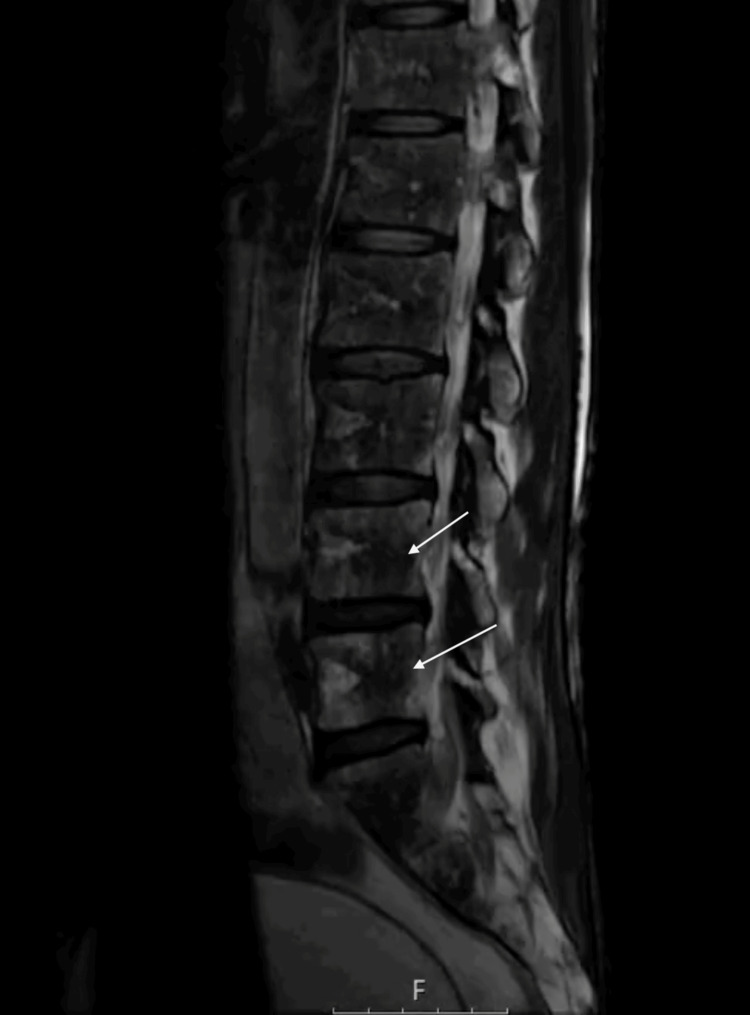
Sagittal T1-Weighted MRI of the Lumbar Spine Sagittal T1-weighted MRI of the lumbar spine demonstrating diffuse low marrow signal intensity across multiple vertebral bodies, reflecting serous (gelatinous) marrow atrophy.
